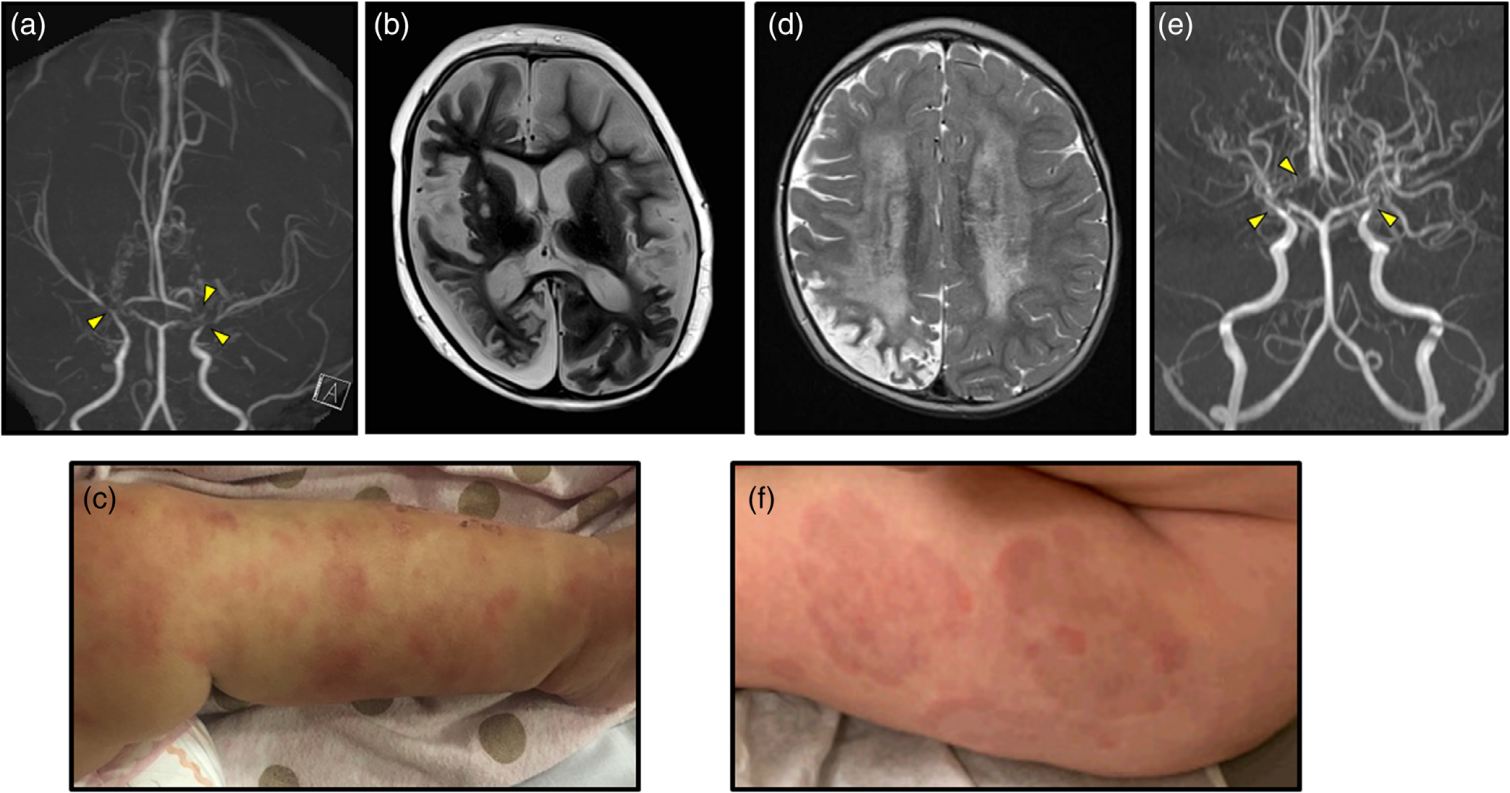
(a) Patient 1 time‐of‐flight MR angiogram technique with 3D reconstructions at 14 months showing marked narrowing of the distal ICA, right middle cerebral artery, bilateral posterior communicating arteries and anterior communicating arteries, and numerous collaterals. (b) Patient 1 T2‐weighted axial MRI at 17 months showing extensive cerebral atrophy and encephalomalacia secondary to recurrent infarction and chronic ischaemia. (c) Skin findings in Patient 1 showing multiple, flat skin lesions, some with central clearing. (d) T2‐weighted axial MRI for Patient 2 at 20 months of age showing bilateral cerebral white matter signal alteration most consistent with ischemic change, and chronic right posterior division middle cerebral artery stroke with marked encephalomalacia and gliosis, consistent with the hypoperfusion state present in a high grade moyamoya (e) Patient 2 time‐of‐flight MR angiogram technique with 3D reconstructions at 20 months showing marked irregularity and minimal to no flow related enhancement within the distal supraclinoid internal carotid arteries, A1 and M1 segments bilaterally, diminished flow related enhancement within the M2 segments and beyond, worse on the right than the left, with marked increase in bilateral lenticulostriate collaterals. (f) Skin findings in Patient 2 showing multiple, flat annular rashes with central clearing [Color figure can be viewed at wileyonlinelibrary.com]
